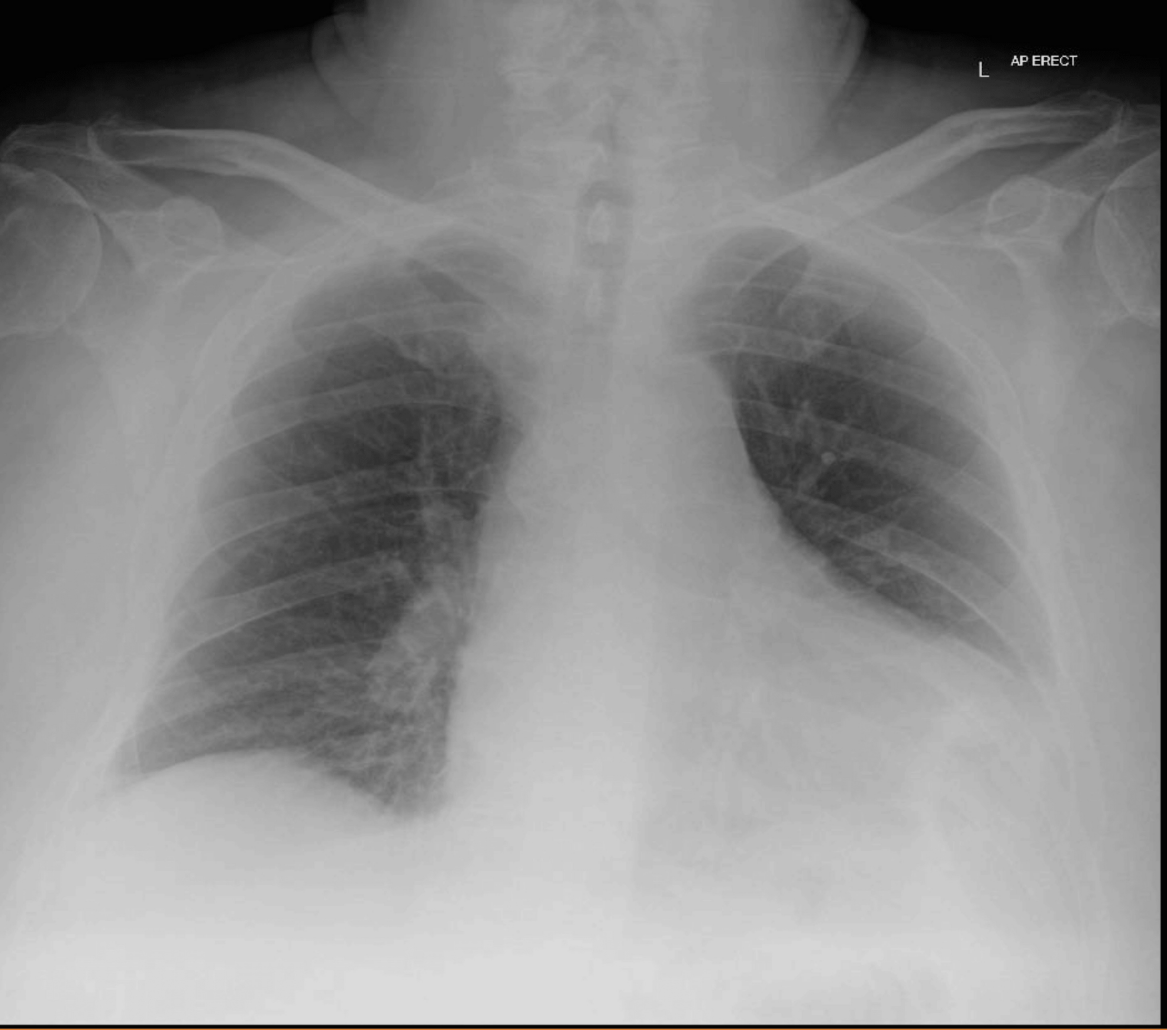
Chest X-ray (AP Erect) Chest radiograph (AP erect) demonstrating a prominent cardiac silhouette, supporting cardiac involvement in the context of immune checkpoint inhibitor-induced neuromuscular toxicity.
